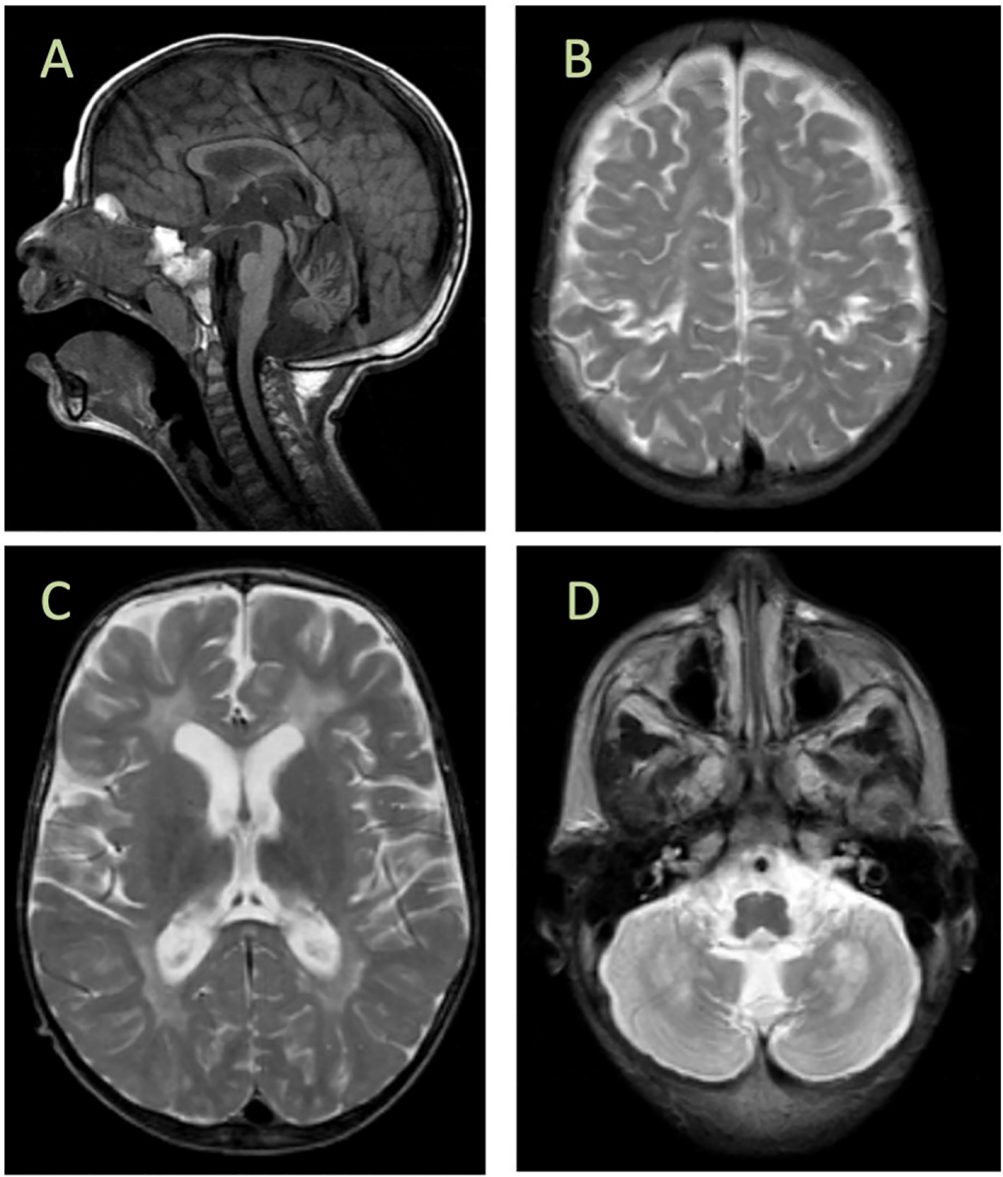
Brain MRI findings. (A) Cerebellar atrophy and decreased signal in the corpus callosum, diffuse increased signal, and cystic encephalomalacia in the white matter (B, C) with bilateral involvement of the gray matter around the central sulcus (D). MRI was performed at age of 2 years.
